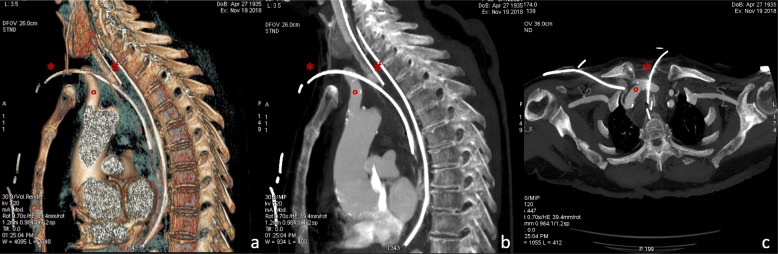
Panel a, sagittal view CT 3-dimensional volume rendering; panel b, sagittal view CT image; panel c, axial view CT image showing the innominate trunk crossed by the guidewire. * guidewire, ° Brachiocefalic trunk, # oro-tracheal tube
